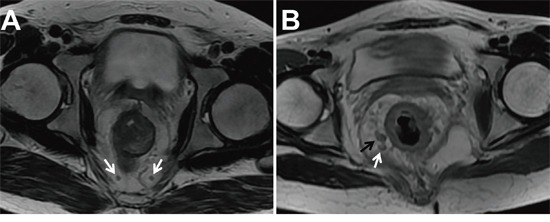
Two T2-weighted axial images in different patients with low rectal cancers A. Two lymph nodes (LN) within mesorectal fascia with the short axis diameter of 2.5 and 3.0 mm, regular borders, and homogenous signal intensity were considered as non-metastatic LNs (white arrow). B. The LN within mesorectal fascia with the short axis diameter of 6.3 mm and mixed signal intensity was considered metastatic (black arrow). Another LN with the short axis diameter less than 5mm (4.2 mm) but with mixed signal intensity was also considered metastasis (white arrow).
